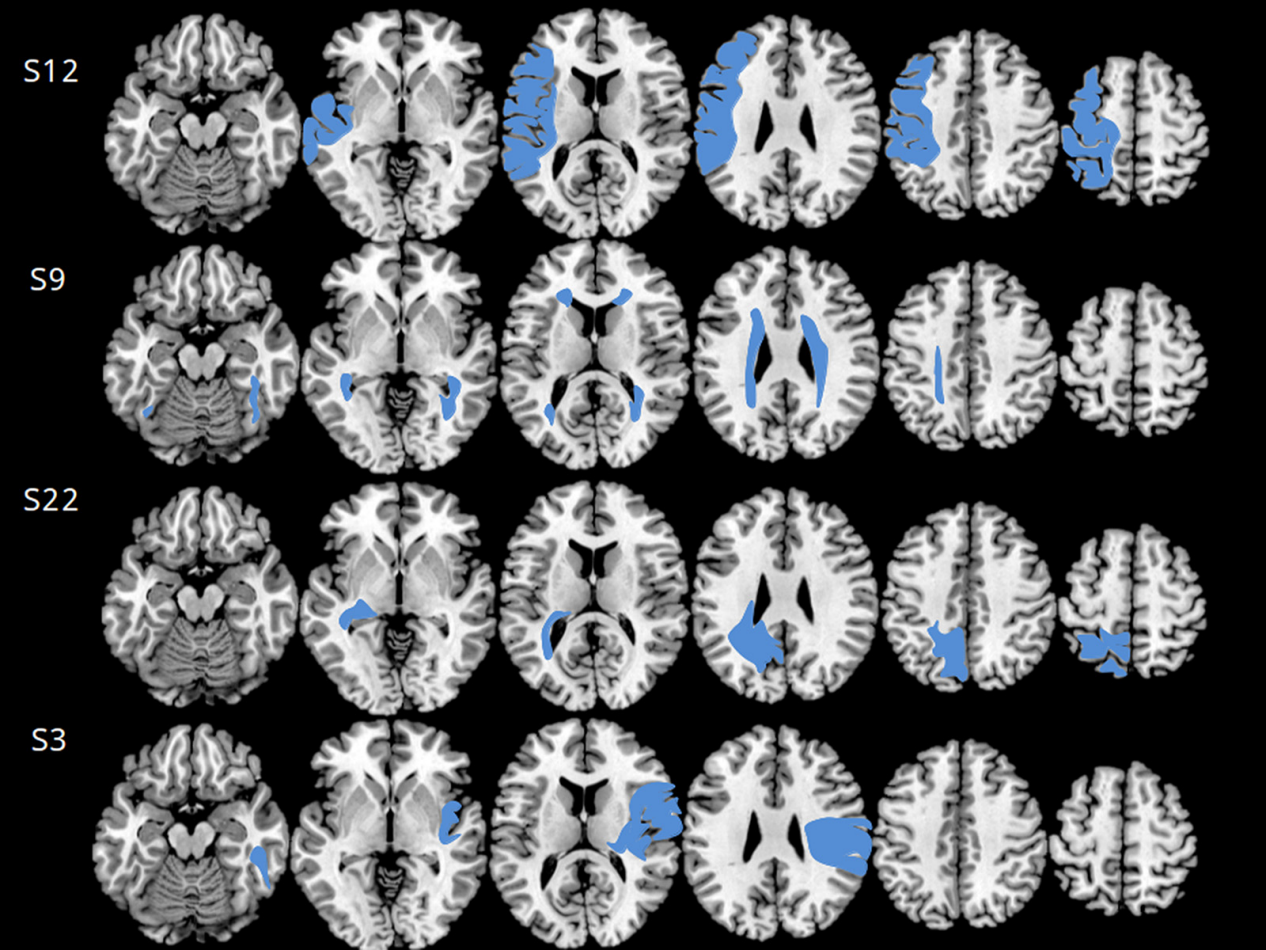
Axial MRI scans used for scoring according to Fiori et al. (2014), relative to four subjects (subject numbers are indicated on the left). The six axial scans best matching the scale template is selected for each subject (see reference and text for further details on the Section “Materials and Methods”). The four cases shown are different from one another with respect to lesion side, type and extension. Subject 12 had an extensive right brain mal-development involving both gray and middle white matter. The lesion extends well into prefrontal cortex. He scored 4 on the Magic Carpet, which is low for age 12, especially for a CBT score of 5.66, and made 4 path-sparing errors out of 5. Subject 9 had a typical bilateral periventricular leukomalacia, extending to middle white matter and to anterior brain regions. She scored 3.66 on the Magic Carpet and 4 on the CBT, both low scores for age 12, and made 2 path-sparing errors out of 3. Subject 22 had a cortical–sub-cortical gray- and white-matter lesion, mostly on the right side but limited to the occipital and parietal lobes. She scored 4 on the MC and 4.66 on the CBT, making only 1 path-sparing error out of 5. Subject 3 had a typical arterial stroke in the territory of the left middle cerebral artery. She scored 3.66 on the MC and 5.33 on the CBT, making only place-sparing errors. The MC score of the latter subject is in line with control data for age 7, and the gap between CBT and MC scores is probably more related to age than to the lesion.
